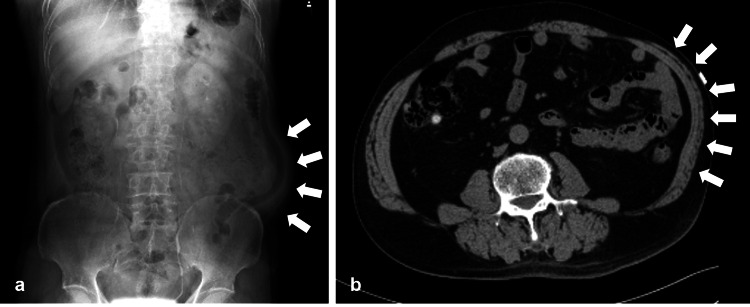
(a) Plain abdominal X-ray: The profile of the left-sided abdominal wall bulging is evident (arrows), but no detailed structure is visible. (b) Abdominal computed tomography without contrast (horizontal section): Thinning of the left-sided abdominal muscles is seen (arrows), but there is no lesion in the intra-abdominal space.
